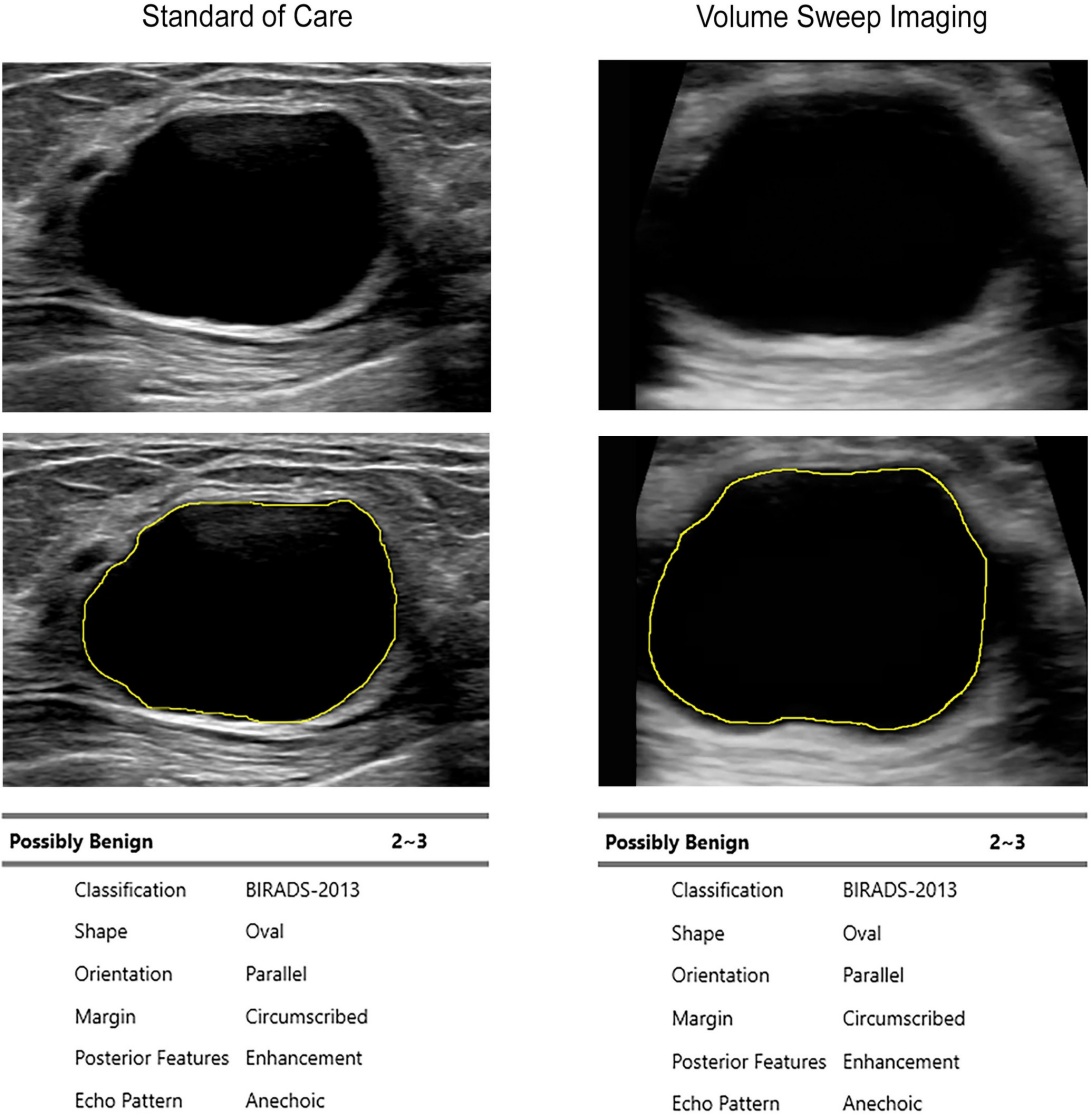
S-Detect Interpretation of a Cyst on Standard of Care and VSI. B-mode ultrasound images of a cyst are shown with their respective mass segmentations and S-Detect produced reports. The standard of care image was obtained by a certified expert breast sonographer using a Philips Epiq 7G ultrasound machine. The VSI image was obtained by a medical student without prior medical experience after less than 2 hours of training on a portable Butterfly iQ ultrasound probe. S-Detect results between standard of care and VSI are identical. The S-Detect results were also identical to expert interpretation of standard of care and VSI outside of mass shape which was called round on expert interpretation (a trivial difference).
